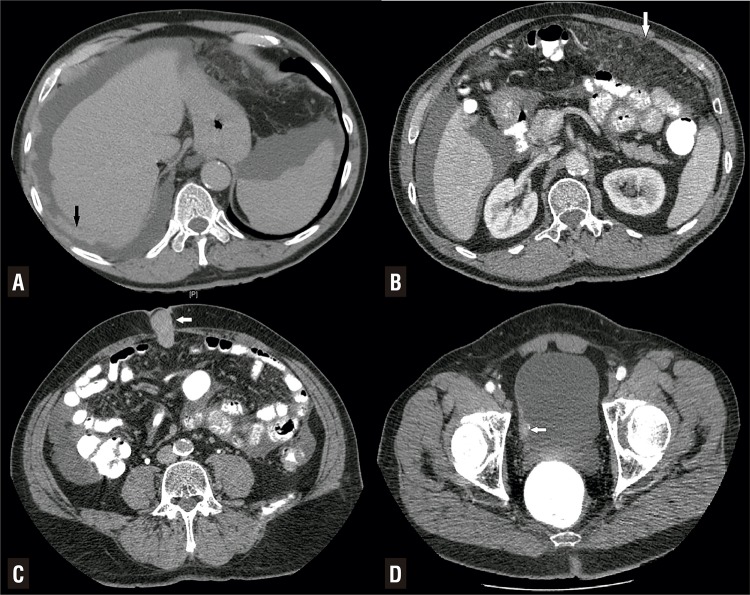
A) Peritoneal thickening on the right hemidiaphragm (black arrow). B) Diffuse omental invasion of peritoneal mesothelioma (omental caking, white arrow). C) CT imaging of umbilical mass (white arrow) D) Bladder mass with sessile growth pattern (white arrow).
